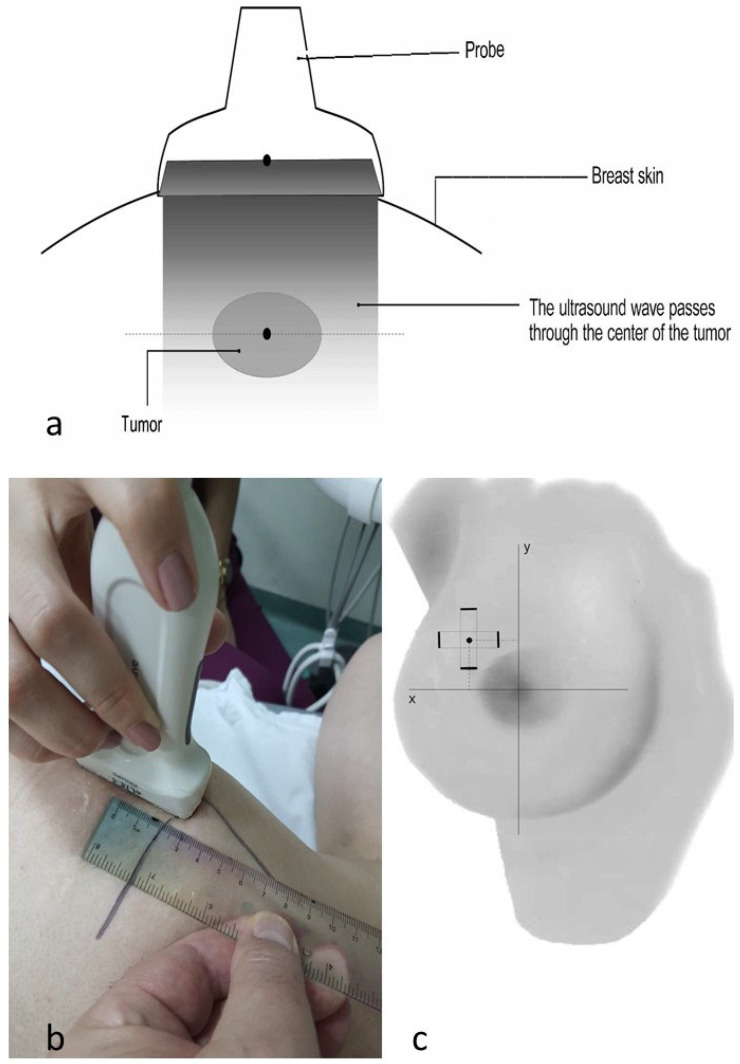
Determining of the projection of the central point of the tumor on the skin. (a) Ultrasound probe placed orthogonally to the surface of the skin above the tumor; the largest section of the tumor is in the center of the ultrasound screen. (b,c) The distances from the center of the ultrasound probe to the corresponding axes drawn on the skin, measured with a ruler; the long axis of the probe in both positions must be absolutely parallel with the “x” and “y” axes.
